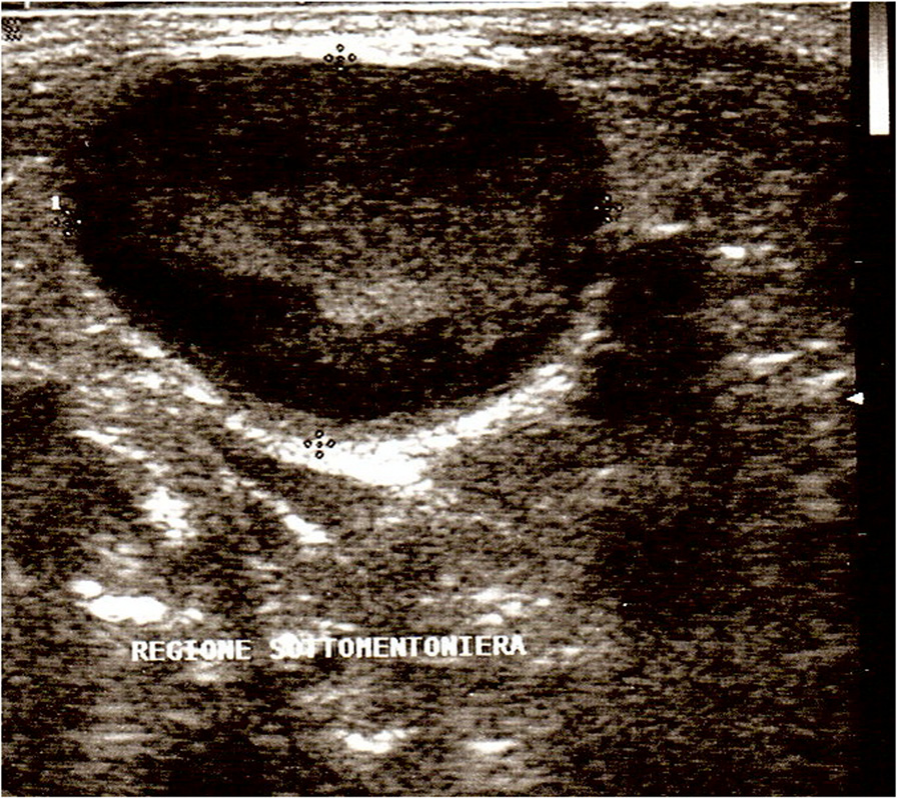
Ultrasonographic findings in an 8-month-old girl with a midline neck lesion. Sonogram shows a well-defined oval mass characterized by a hypoechoic peripheral layer and heterogeneous internal echoes.
